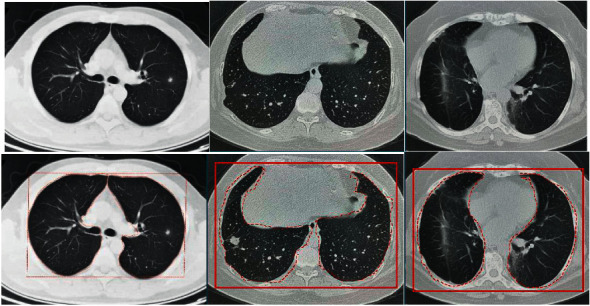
Comparison of the segmentation effect of Mask-RCNN algorithm on lung CT images (the box represents the automatic selection and labeling of lung parenchyma by Mask-RCNN algorithm, and the dotted line represents the further automatic segmentation of lung parenchyma).
